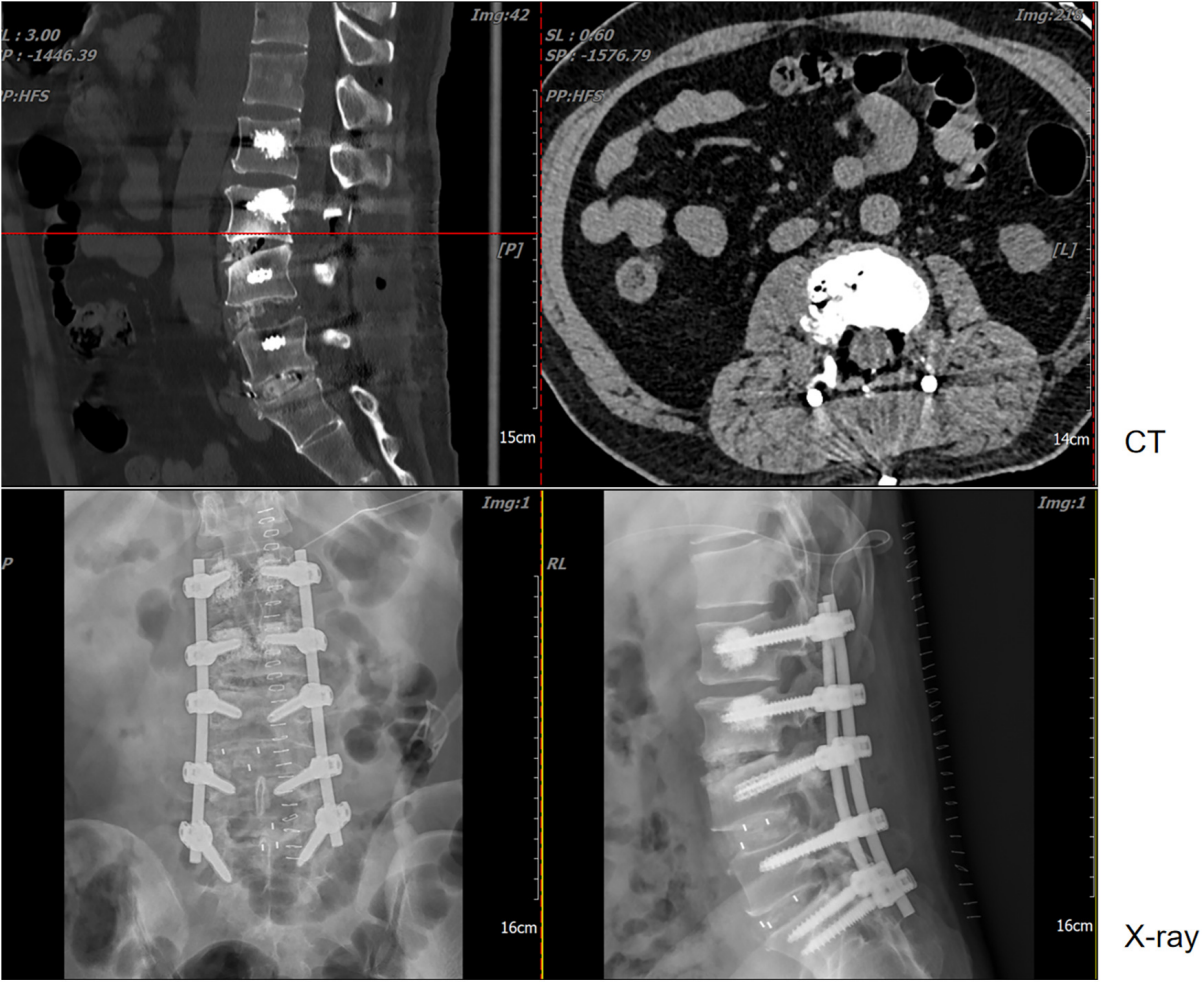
Postoperative CT and anteroposterior and lateral x-ray images.
